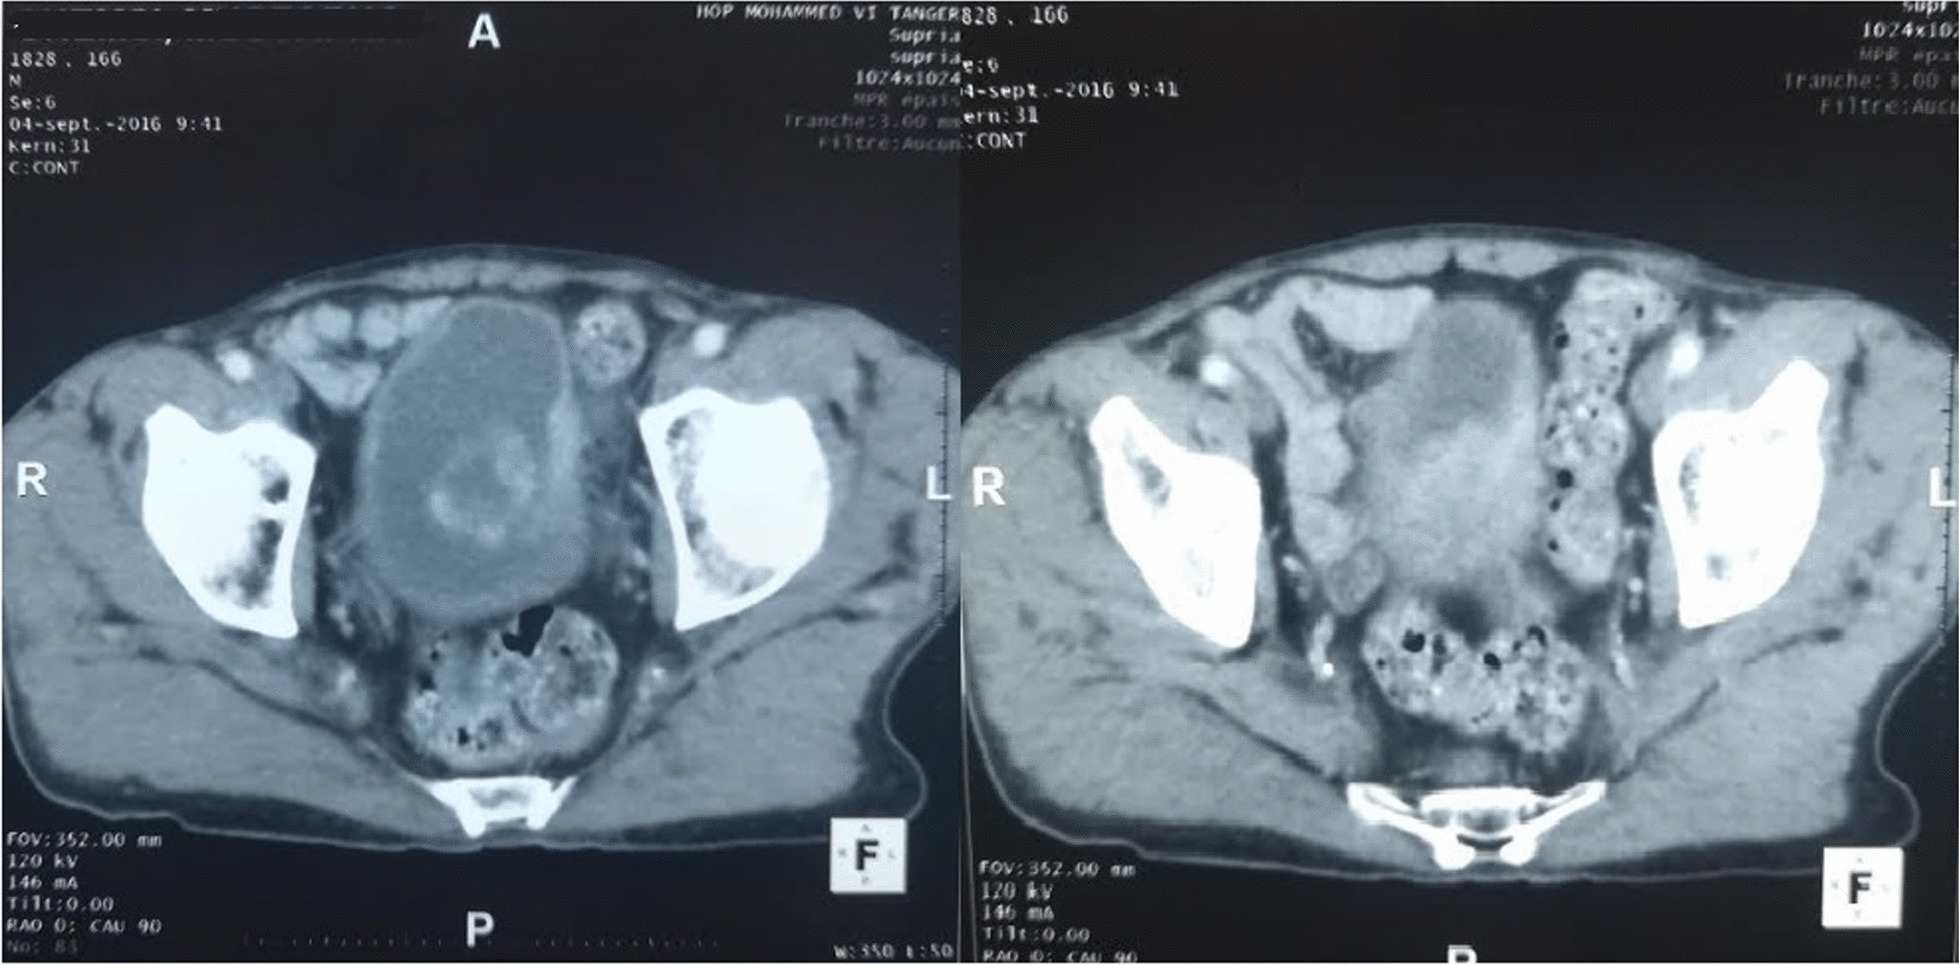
Pelvic computed tomography scan after injection of contrast showing an heterogeneous mass, invading the bladder wall until serosa
